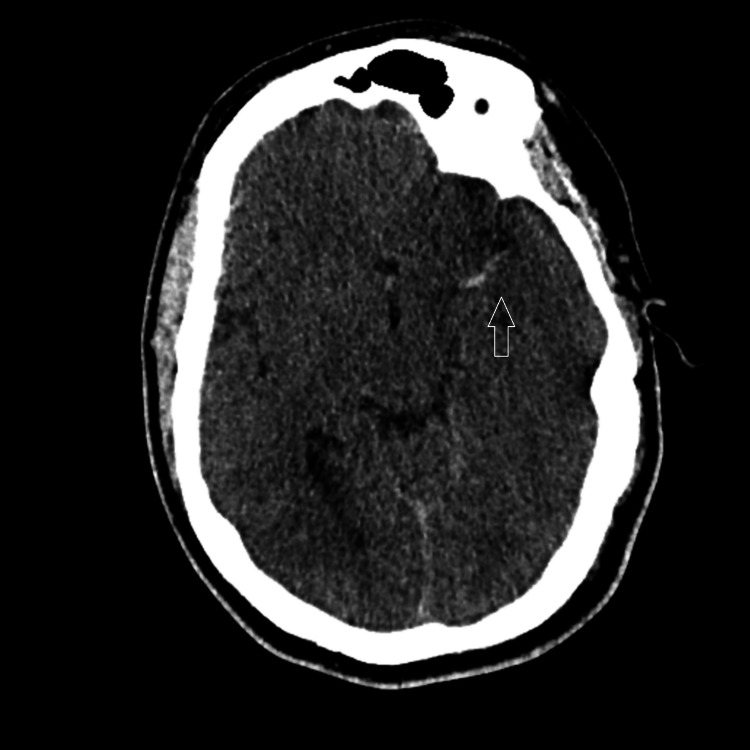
CT brain plain-axial film. Hyper-dense left MCA sign and loss of grey-white matter differentiation
